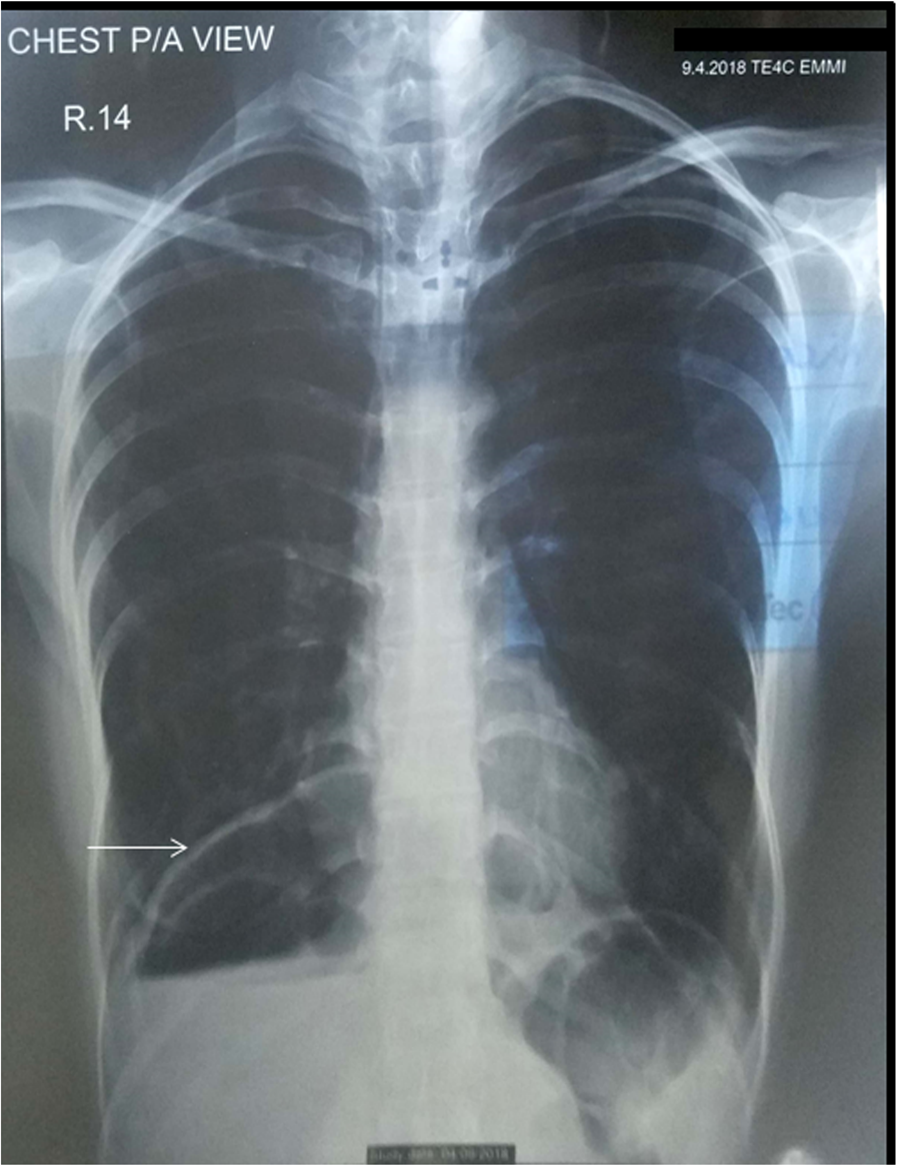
Plain postero-anterior chest X-ray showing abnormal air fluid level (white arrow) in the right basal hemi-thorax above the diaphragm
